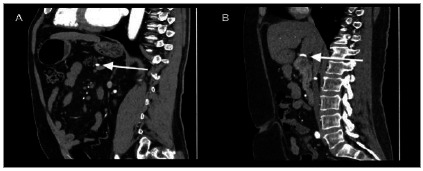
Computed tomography sagittal plane reconstructions demonstrating (A) dorsal course (white arrow) and (B) suprapancreatic course (white arrow) of the splenic artery.
